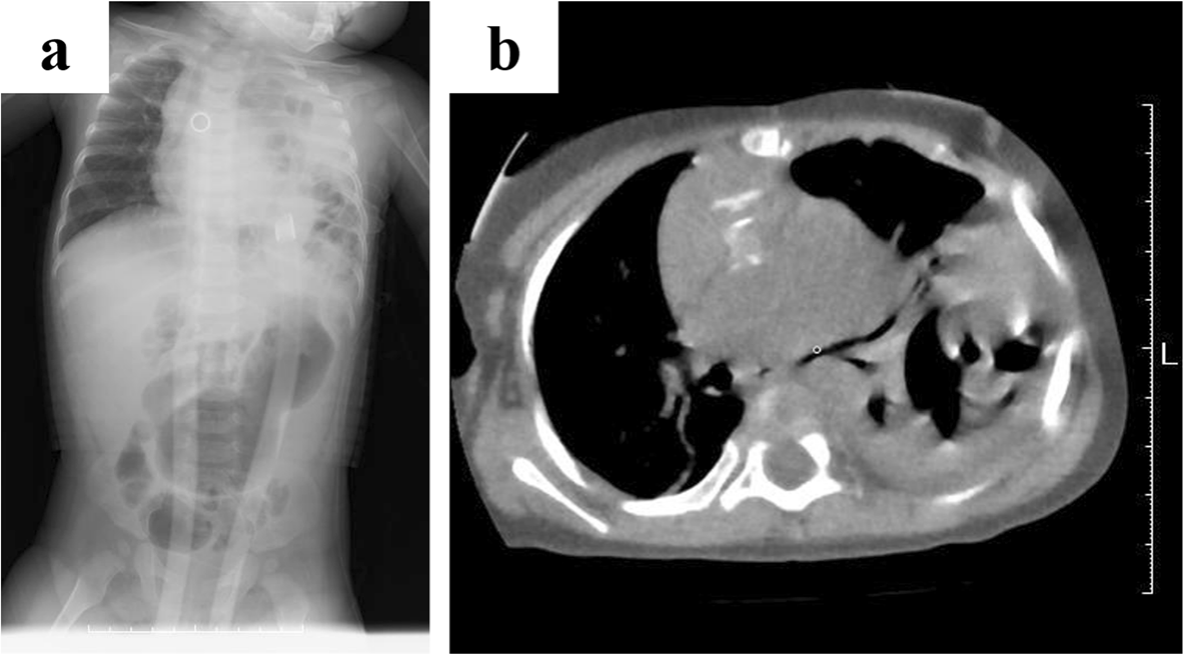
Preoperative radiological findings. a Plain X-ray shows suspected bowel in the left thorax. b Computed tomography confirmed diaphragmatic hernia
